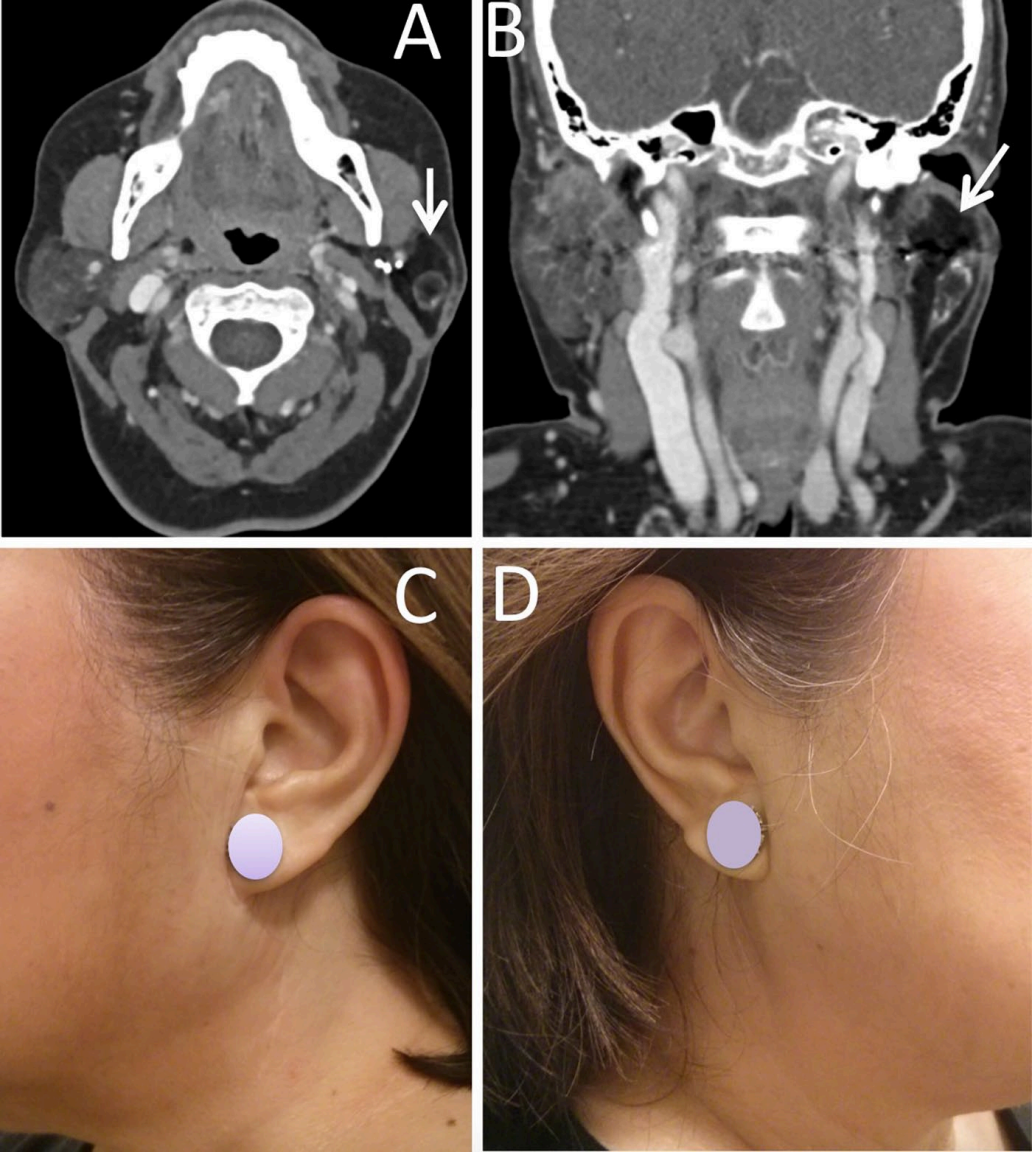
Dermal-fat-fascia graft at 4.5 years following reconstruction for a left lateral parotidectomy defect for a pleomorphic adenoma in axial (A) and coronal (B) CT scan imaging. (C) Facial contour of the operated side. (D) Facial contour of the non-operated side.
